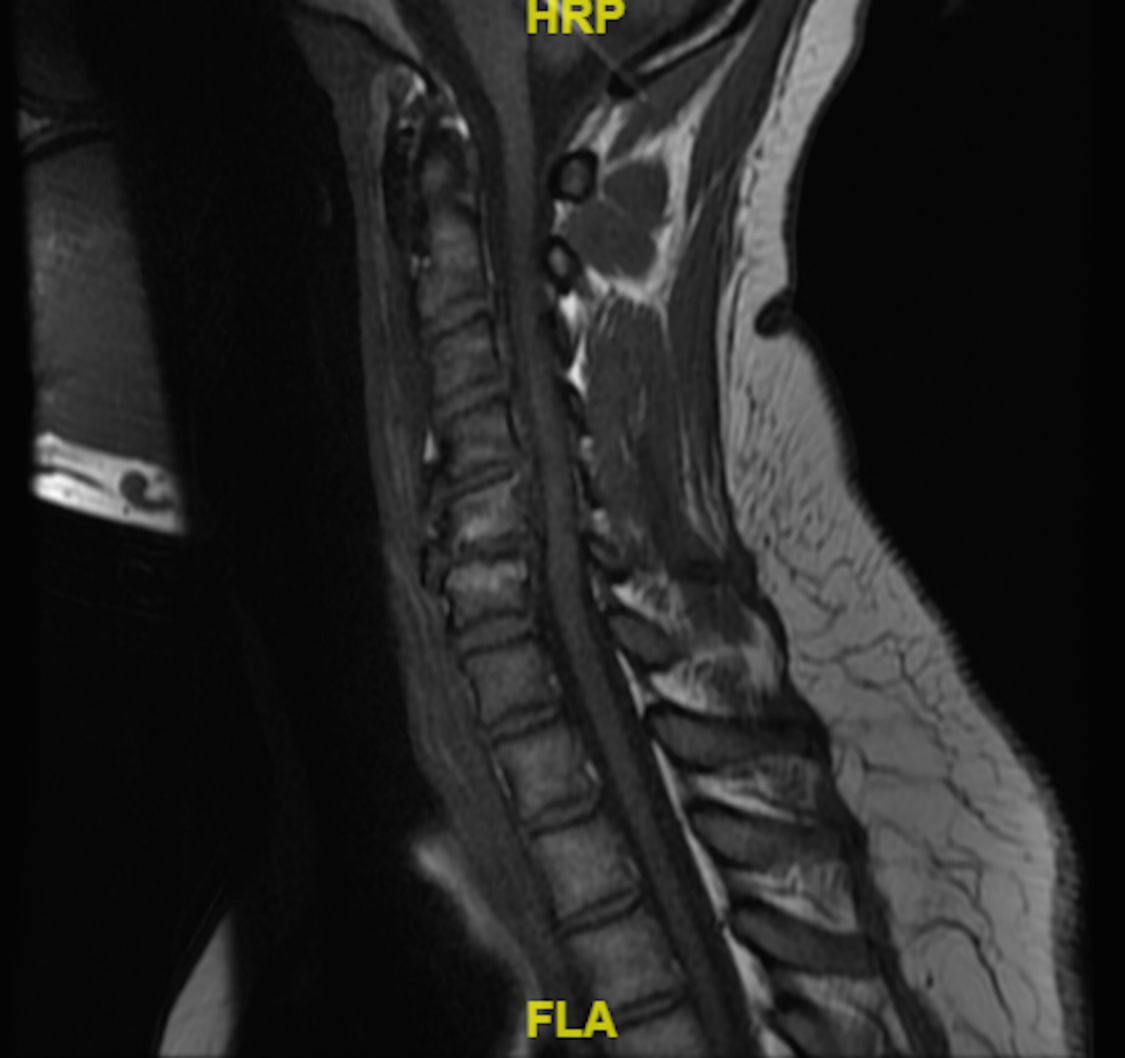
Sagittal view of MRI of the cervical spine. Note that the calcifications are not as well appreciated when compared to CT
